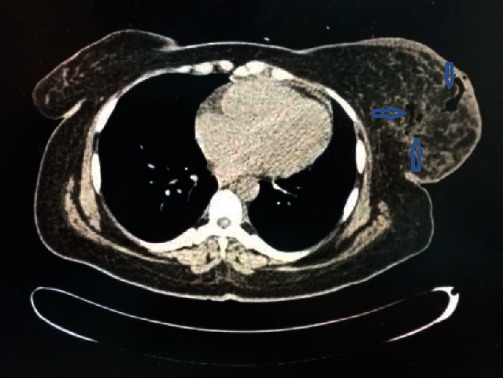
CT scan of the chest showing gas within the left breast (see blue arrows).
